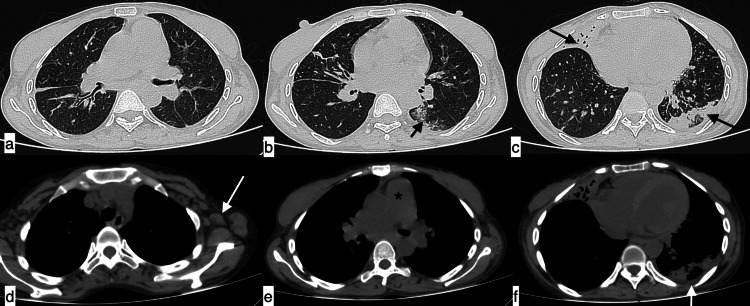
Infective aetiology - community-acquired pneumonia A 31-year-old female patient with SLE presented with complaints of fever, cough, chest pain, and shortness of breath of seven-day duration. Axial HRCT chest images in the lung window (a-c) revealed patchy areas of consolidation (black arrows) in the right middle lobe and basal segment of the left lower lobe with minimal left synpneumonic pleural effusion (f). Additionally, an enlarged pulmonary artery (e, asterisk), cardiomegaly, and axillary lymphadenopathy (d, white arrow) were observed in mediastinal window images. Features were suggestive of infective aetiology, which was confirmed clinically as community-acquired pneumonia. SLE: systemic lupus erythematosus; HRCT: high-resolution CT
